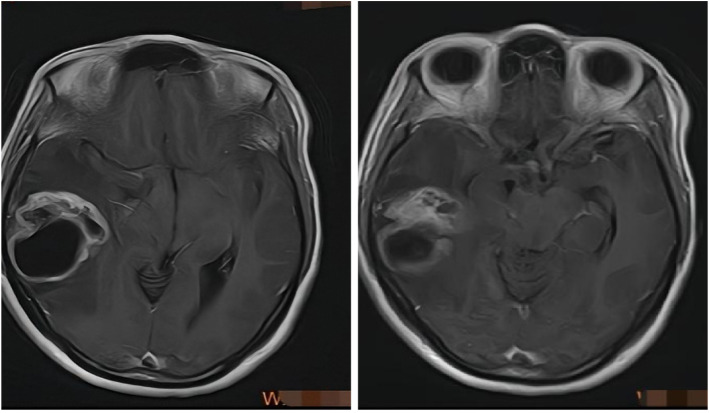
T1-weighted sagittal MRI showed an enhancing lesion in the right temporal lobe with enhancing signals.
